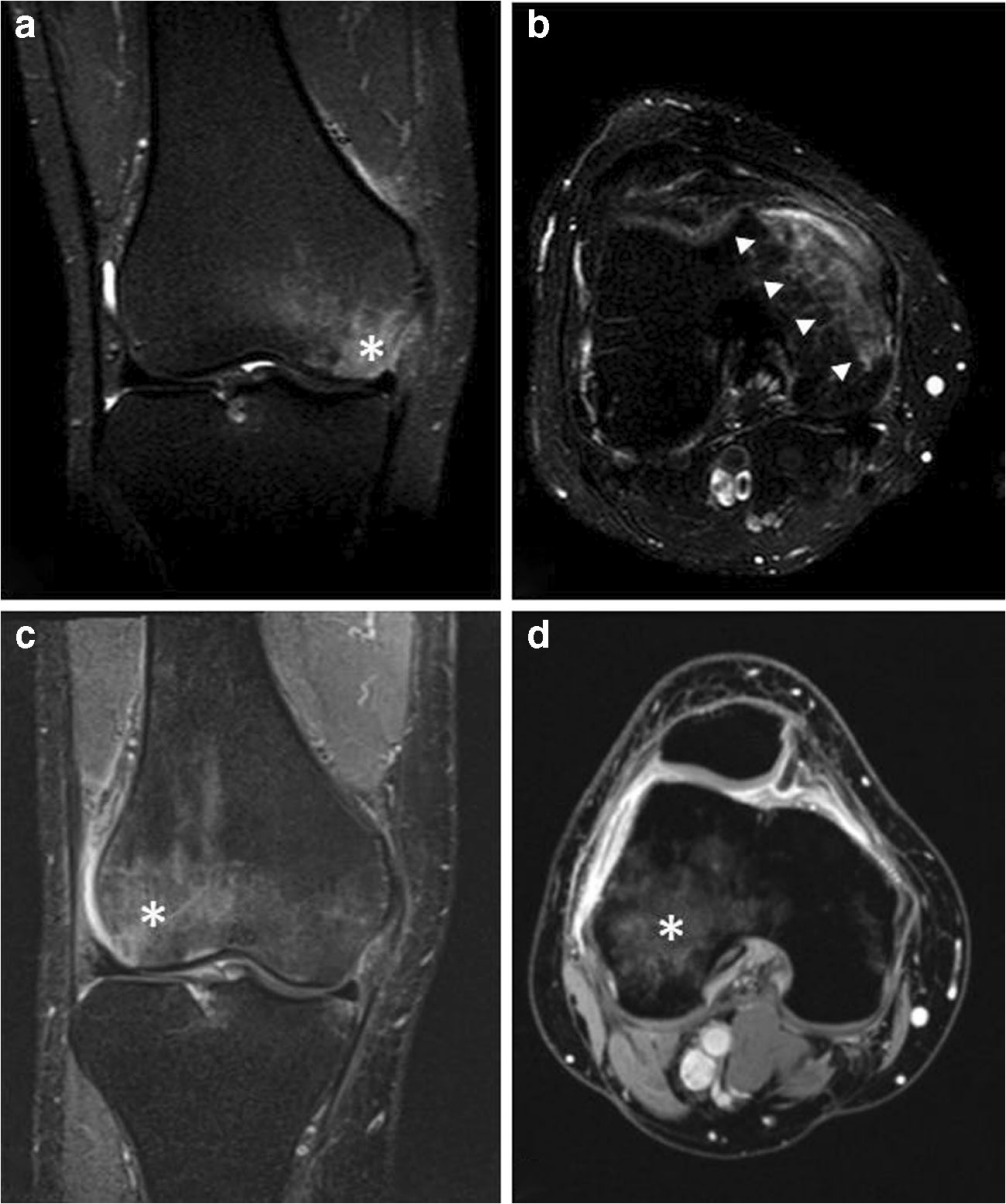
a, b, c, d Primary. A 53-year-old male patient with pain, swelling, and stiffness of the left knee. STIR imaging from the first MRI exam (a, b) highlights edema (A single asterisk (*) in a and arrowheads in b) in the medial condyle; degenerative phenomena affecting meniscuses, articular cartilage and ligaments are not present. STIR imaging performed after two months (c, d) shows that edema (*), from the medial compartment of the knee, has moved into the lateral compartment of the same knee, while all the other findings observed at the first examination remained unchanged. Every possible etiology has been excluded: this is an example of migratory primary ELMSI
